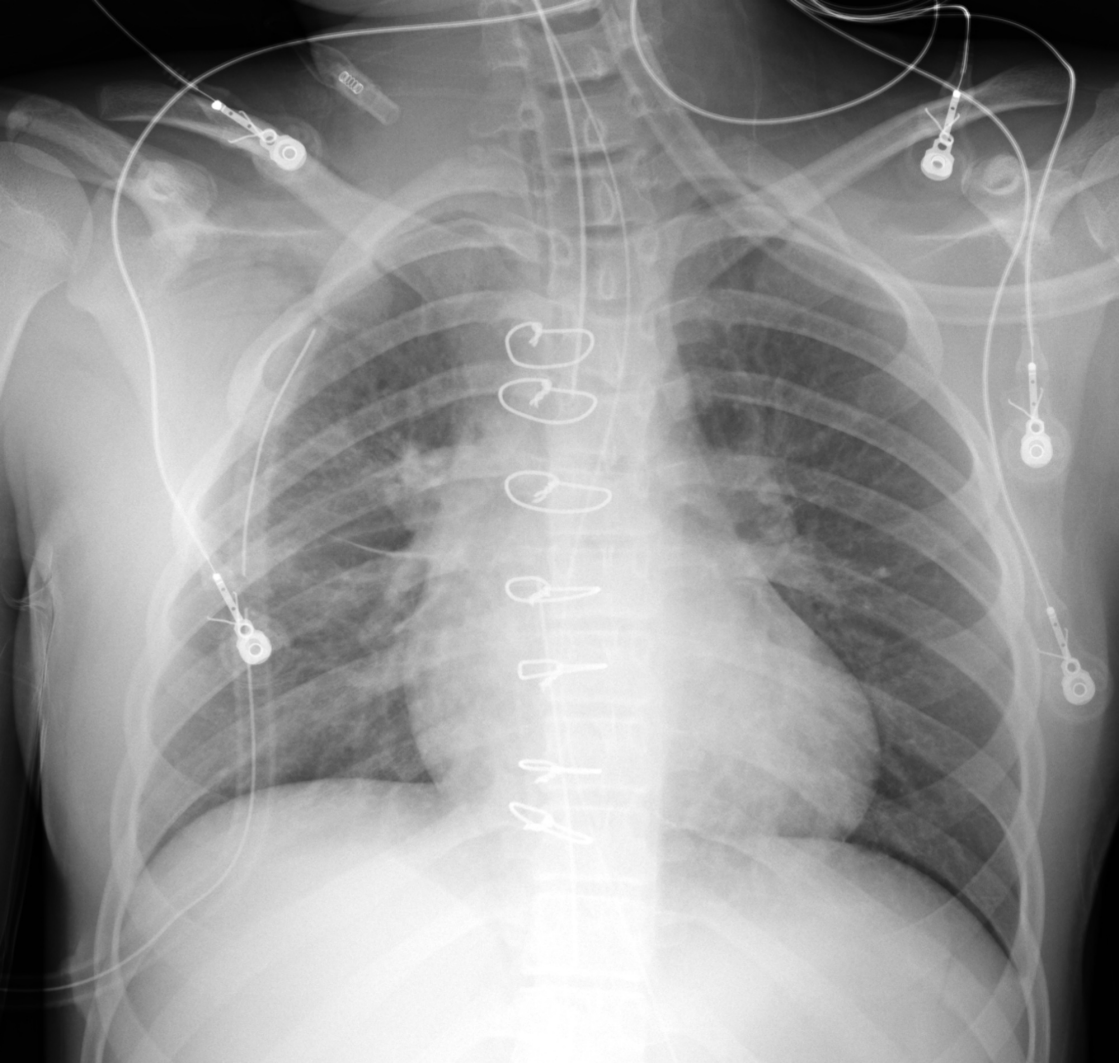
Postoperative frontal radiograph demonstrates interval postsurgical changes of the removal of a large foreign body with midline sternotomy wires, right thoracostomy tube projecting in the right apex, and midline mediastinal drain. The endotracheal tube is seen approximating the carina.
